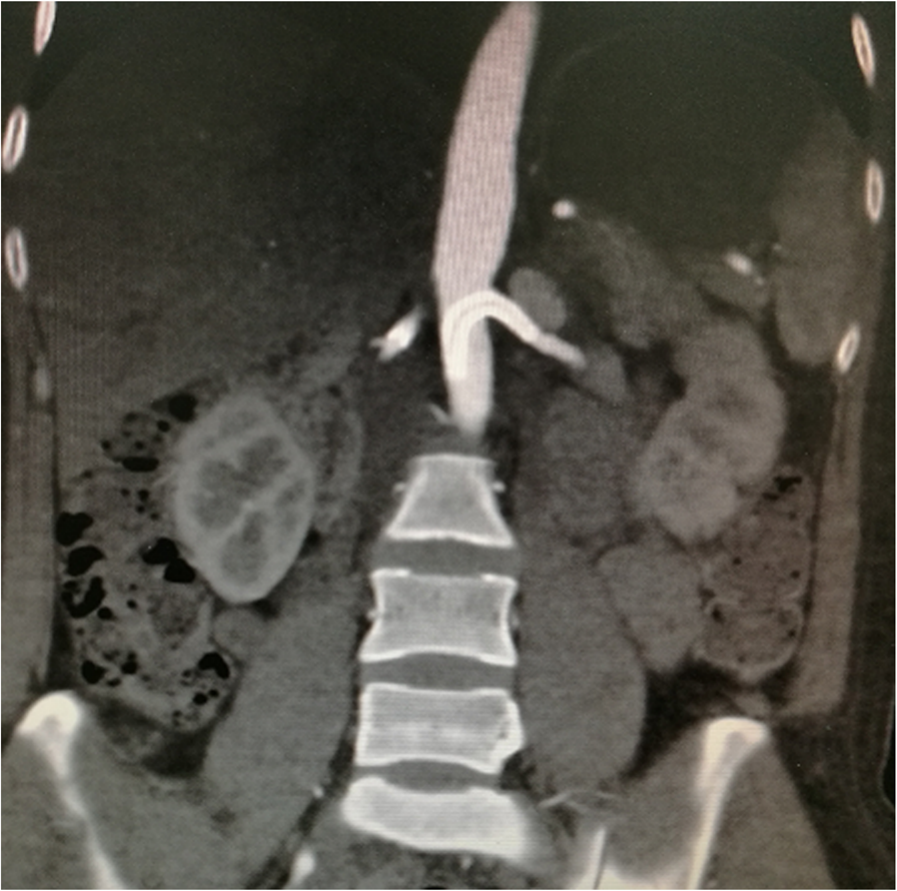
Reconstructed CT revealed the left covered renal artery stent stretching into the aorta
